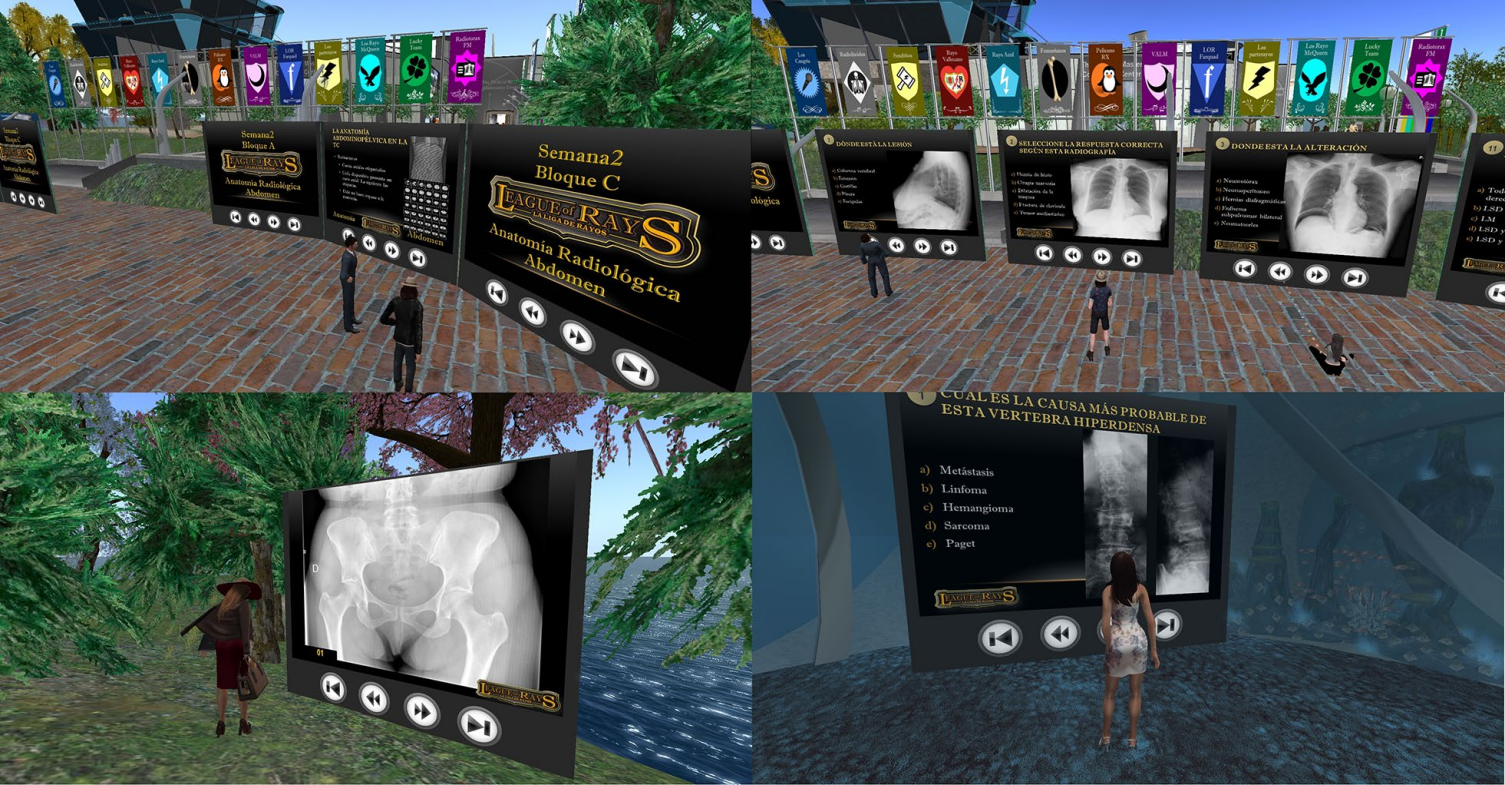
Different scenes during the League of Rays game. Top left: students viewing a set of three learning panels on radiographic anatomy of the abdomen. Top right: Students in front of panels with weekly tests on the central esplanade. Bottom left: a student in front of an N/P task located between the trees. Bottom right: student conducting a weekly test at an underwater facility
